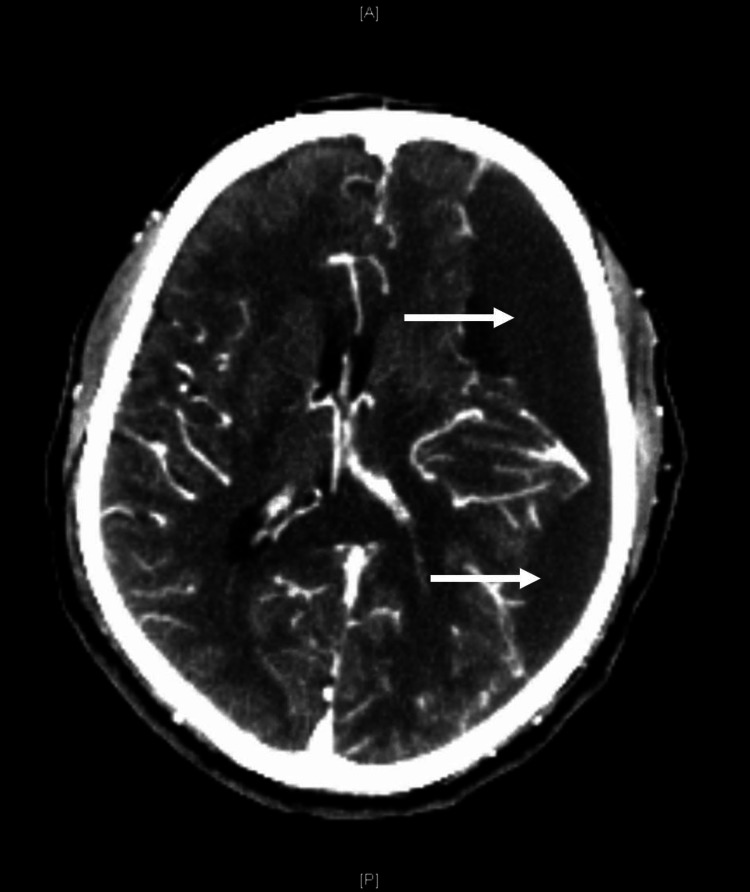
Contrast CT of the head reveals subacute left frontotemporoparietal subdural hematoma. CT: Computed tomography.
